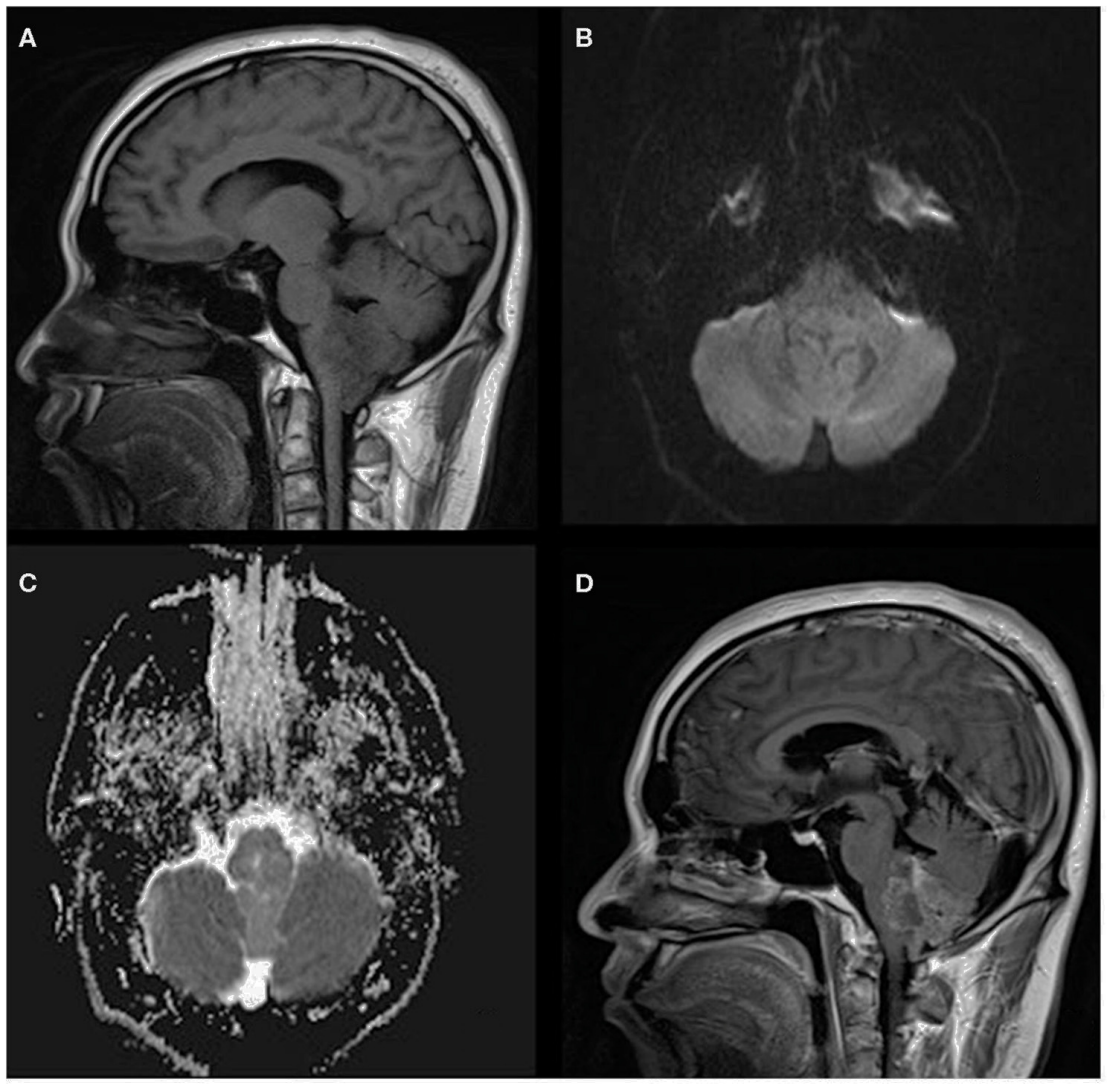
A 33-year-old man with infratentorial anaplastic ependymoma in the fourth ventricle on (A) T1-weighted image, (B) diffusion-weighted images, (C) apparent diffusion coefficient, and (D) contrast T1-weighted image. The tumor appears as heterogeneous signal intensity, small cysts, non-reduced diffusion within the soft tissue component, and absent peritumoral edema.
